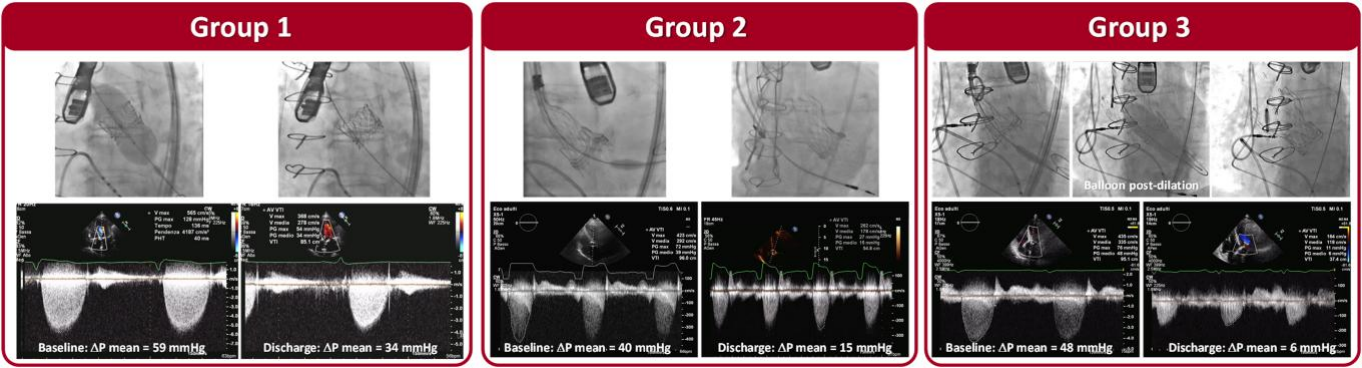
Representative cases. Fluoroscopy and continuous-wave Doppler tracings showing mean pressure gradient at baseline and discharge in a patient from Group 1 (Left Panel), Group 2 (Central Panel), and Group 3 (Right Panel).
